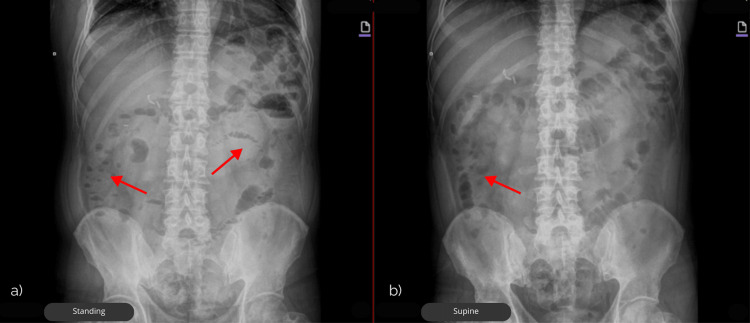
Plain abdominal X-rays in two projections showing short air–fluid levels and the “string of pearls” sign, as indicated by the red arrows in (a) standing and (b) supine positions, suggestive of intestinal obstruction.
